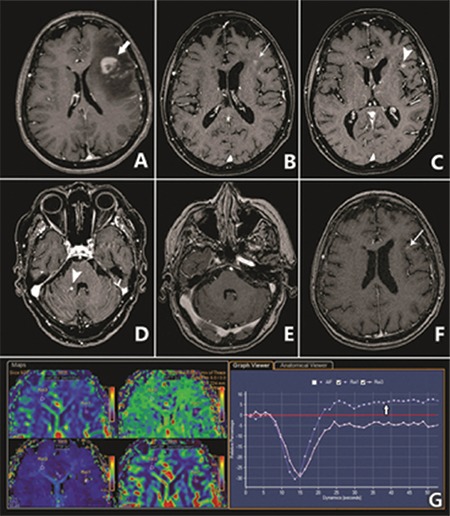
A) Initial presentation of the enhancing lesion in the left frontal lobe (thick arrow), with considerable perilesional edema. B, C, D) After one and four rituximab plus a high-dose methylprednisolone cycles there was a reduction of the enhancing lesion (thin arrow) and edema; however, new enhancing lesions appeared in the left frontal operculum and the right middle cerebellar peduncle (arrowheads). E) Brain magnetic resonance imaging 5 months after ibrutinib therapy demonstrates complete resolution of the cerebellar lesion and F) minimal enhancement in the area of the lesion in the left frontal operculum (arrow). G) Dynamic susceptibility contrast perfusion imaging. Comparison between the enhancing lesion and the normal contralateral side demonstrates an overshooting of the intensity curve of the lesion above the baseline (arrow). This phenomenon is suggestive of lymphoma [149x172 mm (72x72 DPI)].
